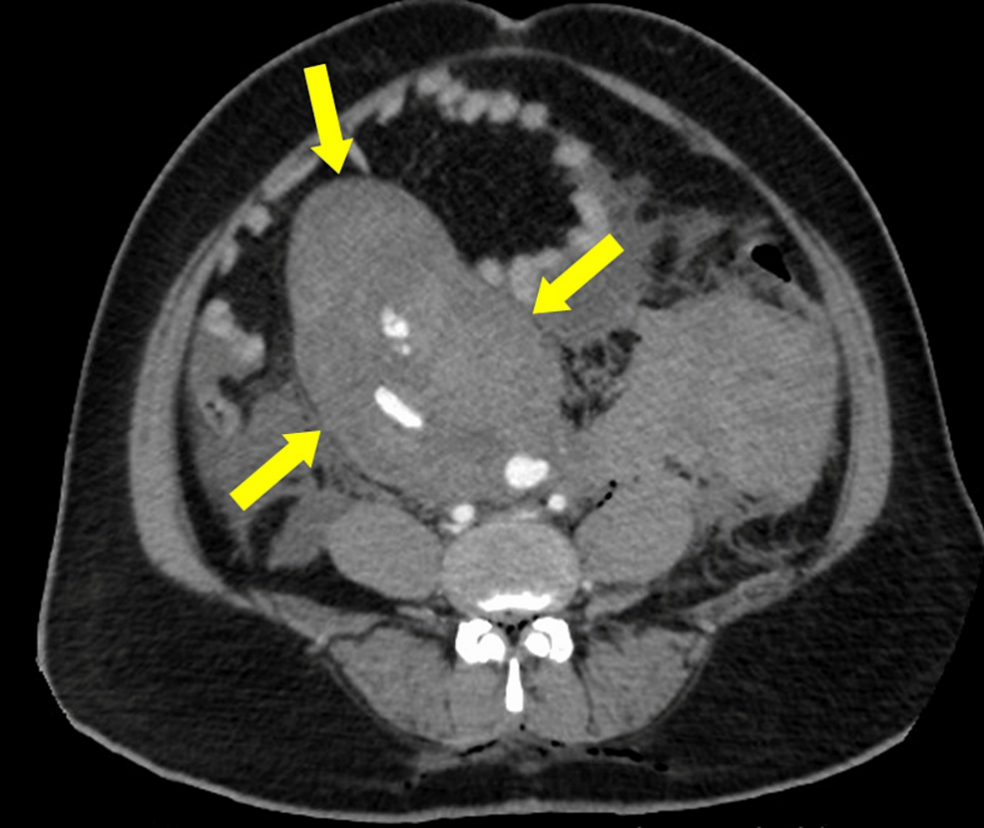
Vascular extravasation and large retroperitoneal hematoma on CTA (axial view). CTA: computed tomography angiography
